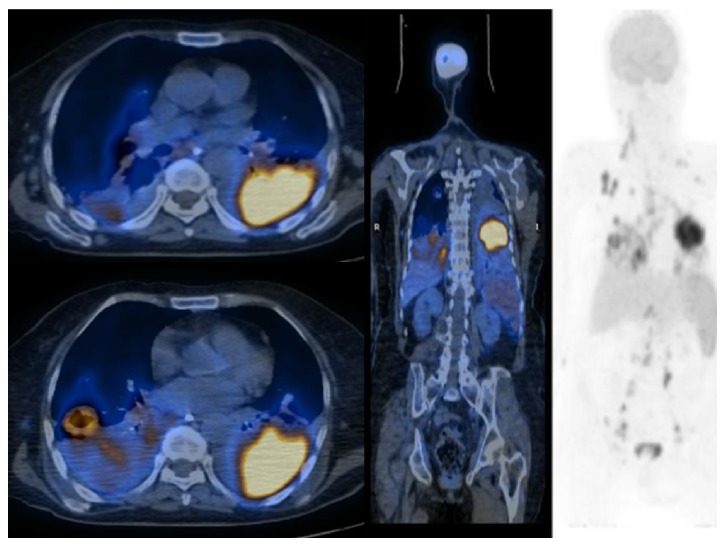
PET/CT scan showing increased 18F-FDG uptake multicompartmental lymphadenopathies and lung lesions.
